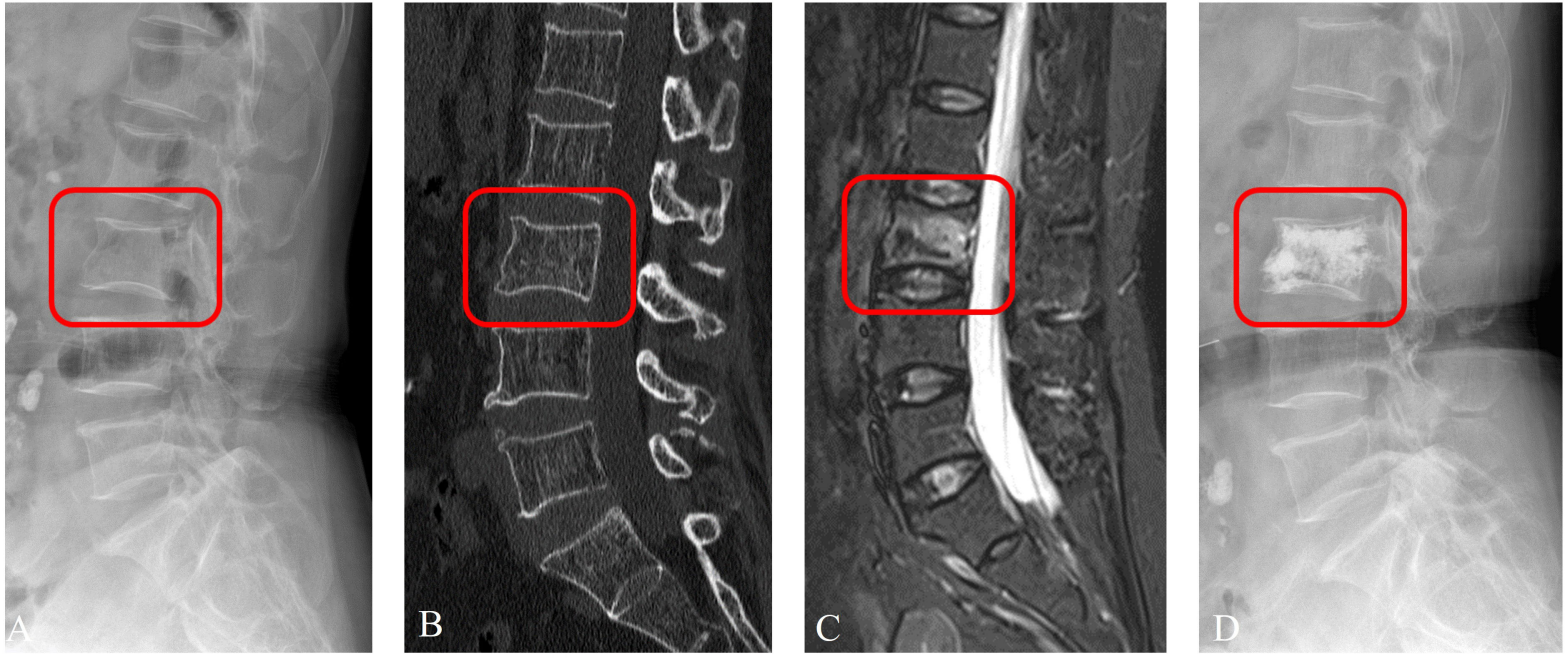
Prediction example based on RadImageNet model. An examples of a 65-year-old female patient with OVFs (ASTLOF score: 5) in the prospective validation set. This case was misclassified by the ImageNet model, but correctly reclassified as the Class 1 by the RadImageNet model (A. X-ray, B. CT, C. MRI, D. X-ray with PVA).
